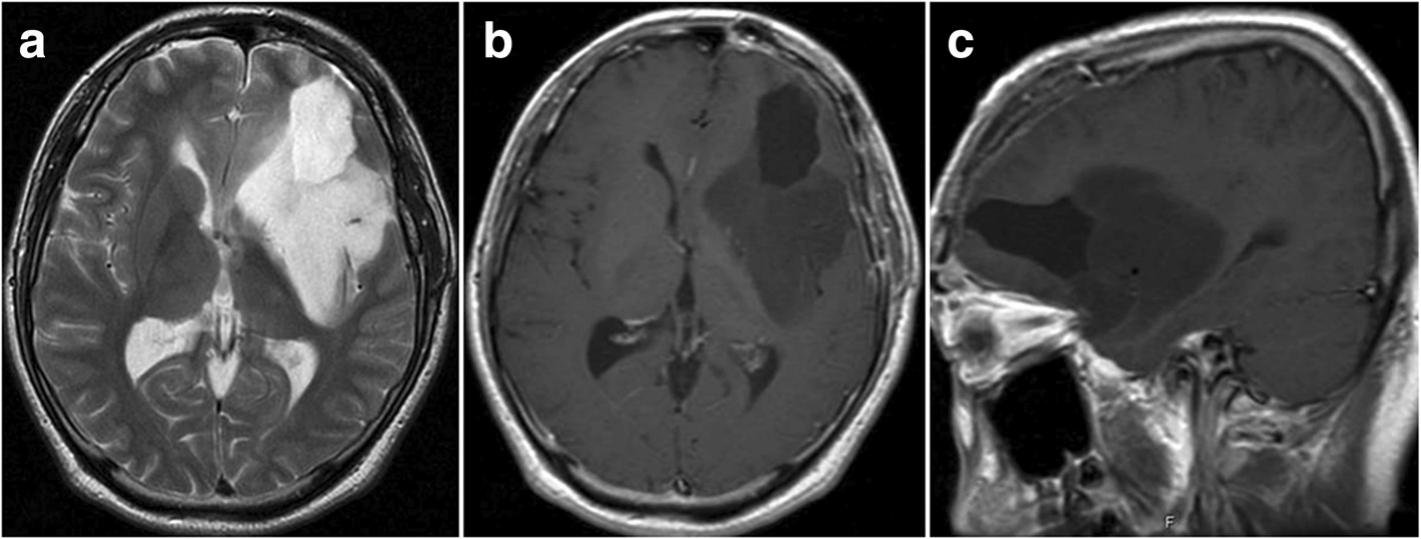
MR images at 3 months after the first surgery showing no growth of the left frontal tumor and no lesion at the other sites including the cerebellum. Axial T2-weighted image (a), axial (b), and sagittal (c) Gd-enhanced T1-weighted MR images
